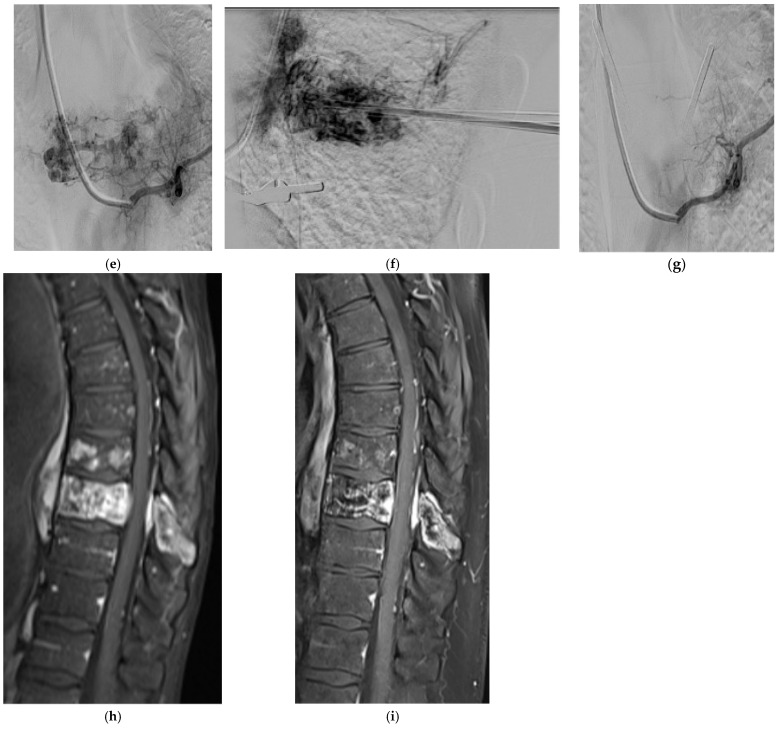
Aggressive hemangioma. (a) Sagittal CT, osseous expansion, and extensive extraosseous extension into the paravertebral soft tissues. (b) Axial CT: osseous expansion results in narrowing of the spinal canal. (c) Sagittal T1 MR: the low signal is unlike that of a typical, nonaggressive hemangioma. (d) Sagittal T2 MR: there is osseous expansion with outward bowing of the posterior cortex of the involved vertebral body. Extraosseous extension is visualized in the prevertebral space. (e) Catheter angiogram with injection in the segmental artery demonstrates prominent vascular channels in the aggressive hemangioma. (f) Fluoroscopic images during liquid embolization of the lesion through a transpedicular approach. (g) Post-procedural catheter angiogram demonstrates marked reduction in vascularity in keeping with successful treatment. (h) Pre-procedural T1 FS contrast-enhanced MR demonstrates the avid enhancement associated with the aggressive hemangioma. (i) Post-procedural follow-up T1 FS contrast-enhanced MR shows marked reduction in enhancement in keeping with interval embolization.
